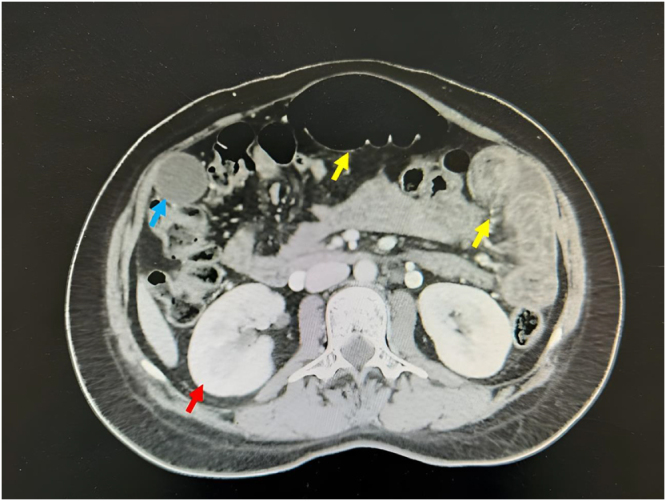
CT scans of the abdomen and pelvis excluded the presence of malignancy or other intra-abdominal pathology (red arrow: kidney, blue arrow: gallbladder, yellow arrows: colon).
